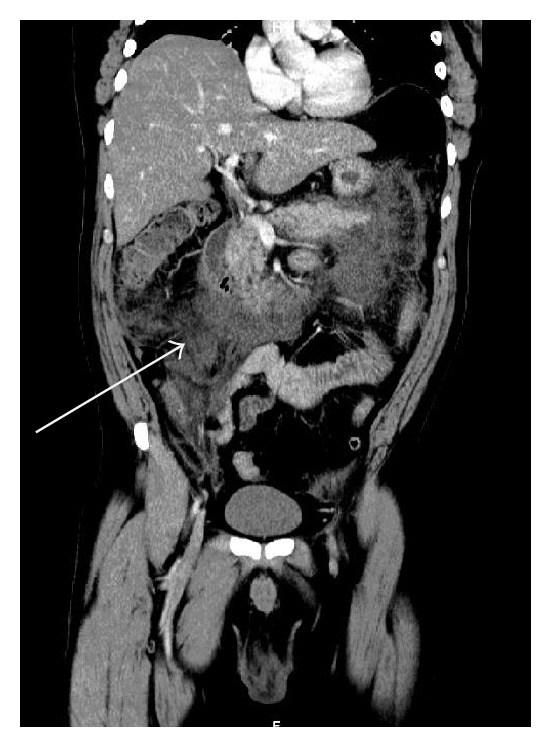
Frontal section contrast CT scan showing retroperitoneal effusion extending from the tail and the body of the pancreas.
